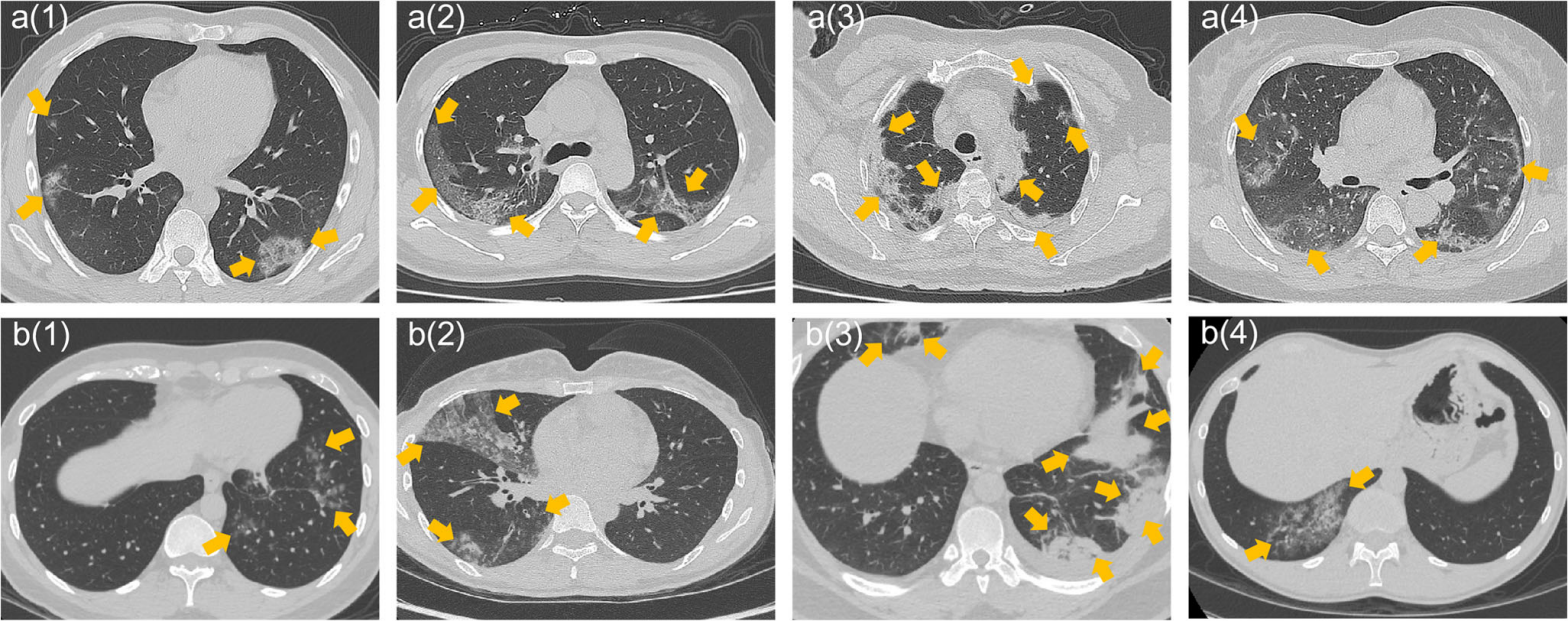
The CT images of COVID-19 positive (a) and COVID-19 negative (b) with significant different signature values based on the combined feature matrix. Figure a(1) represents a 35-year-old male and CT manifested as bilateral opacities, and linear signature score of 1.32 and Lasso signature score of 0.99; figure a(2) denotes a 43-year-old female and CT manifestation are bilateral ground-glass opacities, vascular thickening, and interlobular septal thickening, with signature scores of 1.23 and 0.99; figure a(3) denotes a 62-year-old male and CT manifestation is bilateral multifocal consolidations. Signature scores are 1.24 and 0.98; figure a(4) represents a 45-year-old female and CT manifested as bilateral peripheral multifocal lesions with signature scores of 1.25 and 0.97; figure b(1) represents a 29-year-old male and CT manifestation is multifocal ground-glass opacities in the left lung. Signature scores are − 0.14 and 0.04; figure b(2) represents a 30-year-old female and CT manifestation is multifocal, mixed ground-glass opacity and consolidation in the right lung. Signature scores are − 0.11 and 0.08; figure b(3) represents a 30-year-old male and CT manifestation is bilateral multifocal consolidation. Signature scores are 0.07 and 0.70; figure b(4) represents a 29-year-old male and CT manifested as mixed densities in the right lung. Signature scores are − 0.17 and 0.03, respectively
